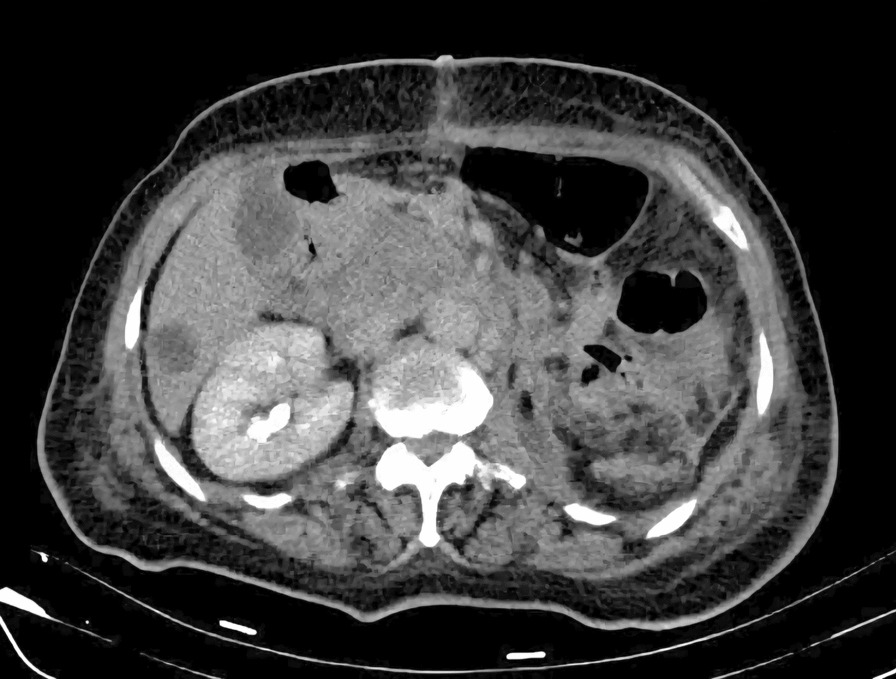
Postoperative computed tomography
